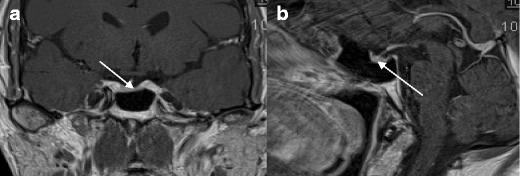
Case 3 (pituitary fibrosis secondary to tuberculous CNS infection). a Coronal MRI showing low volume pituitary gland. b Sagittal MRI showing low volume pituitary gland and probable pituitary fibrosis
